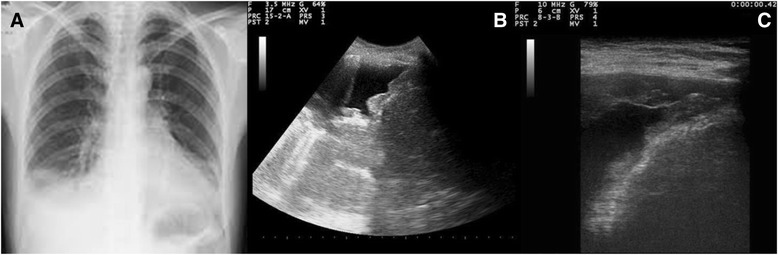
a: patient with right pleural effusion, plain chest x-ray. b and c: Bedside Chest ultrasonography strategically revealing nodular variations of the parietal pleura, diaphragm and costophrenic sinus. This serendipitous finding immediately orients towards the most likely diagnosis (neoplastic pleural involvement)
